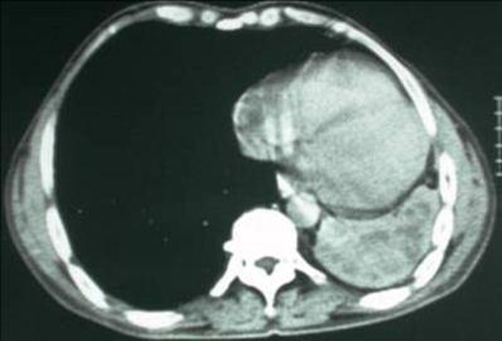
Computed tomography (CT) scan showing multiple cystic cavities in the left lung.
